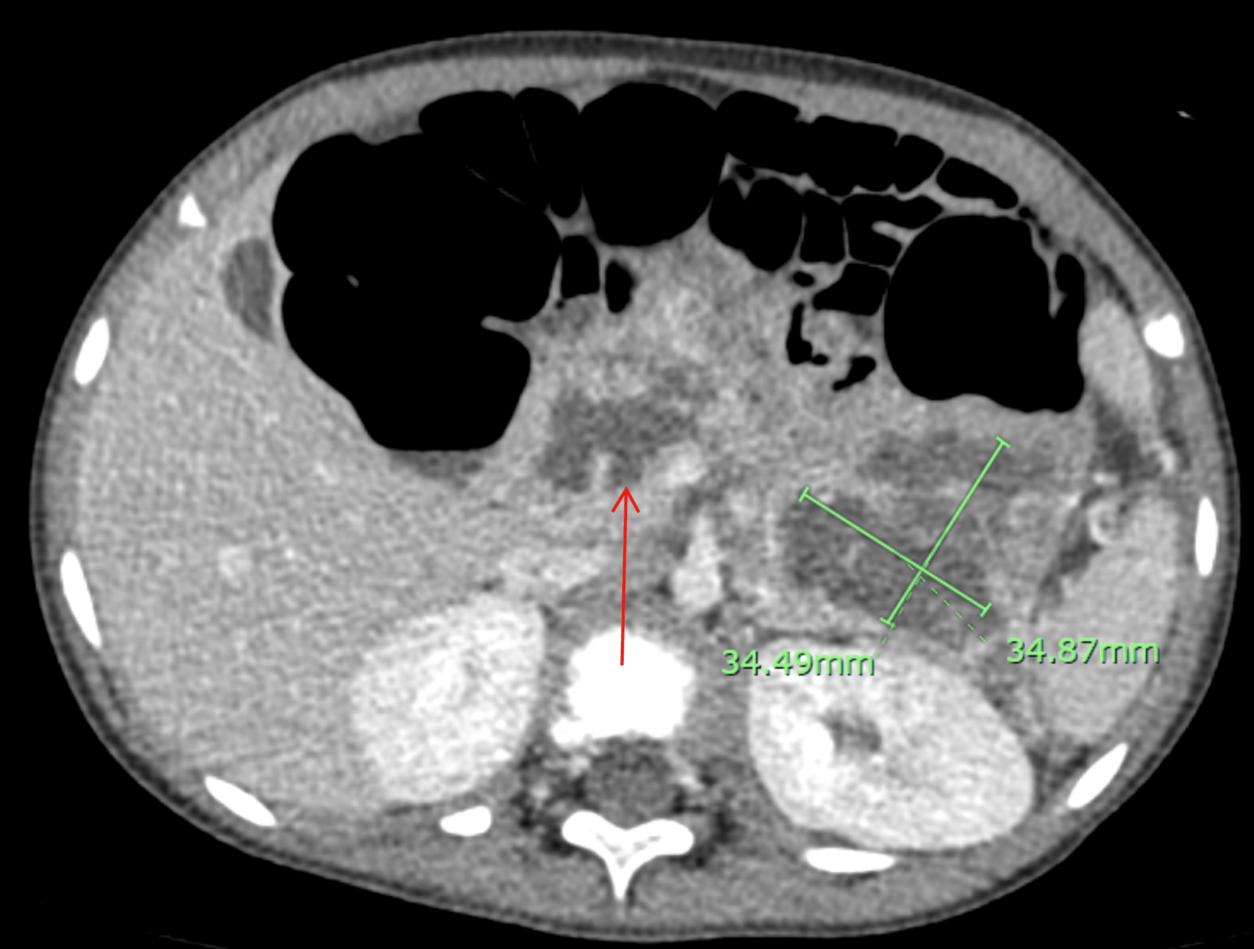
CT abdomen & pelvis with IV contrast revealing the remainder of the pancreas is almost entirely replaced by a loculated fluid collection without gas extending from the pancreatic head (red arrow) to the tail (green measuring marker), with either enhancement of parenchyma or peripancreatic tissue. This measures approximately 8 cm in length by 2 cm anteroposterior by 3.5 cm craniocaudal.
